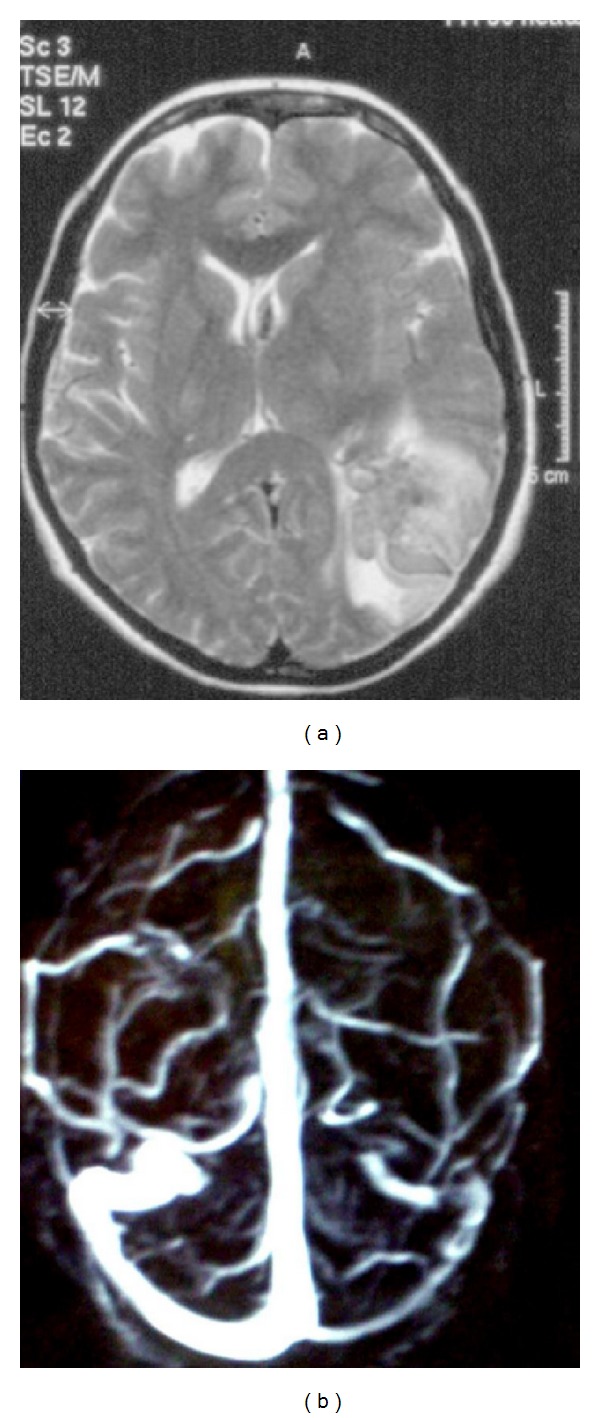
Patient 2: MRI showed a left temporal hematoma while the MRV revealed a left TS thrombosis.
